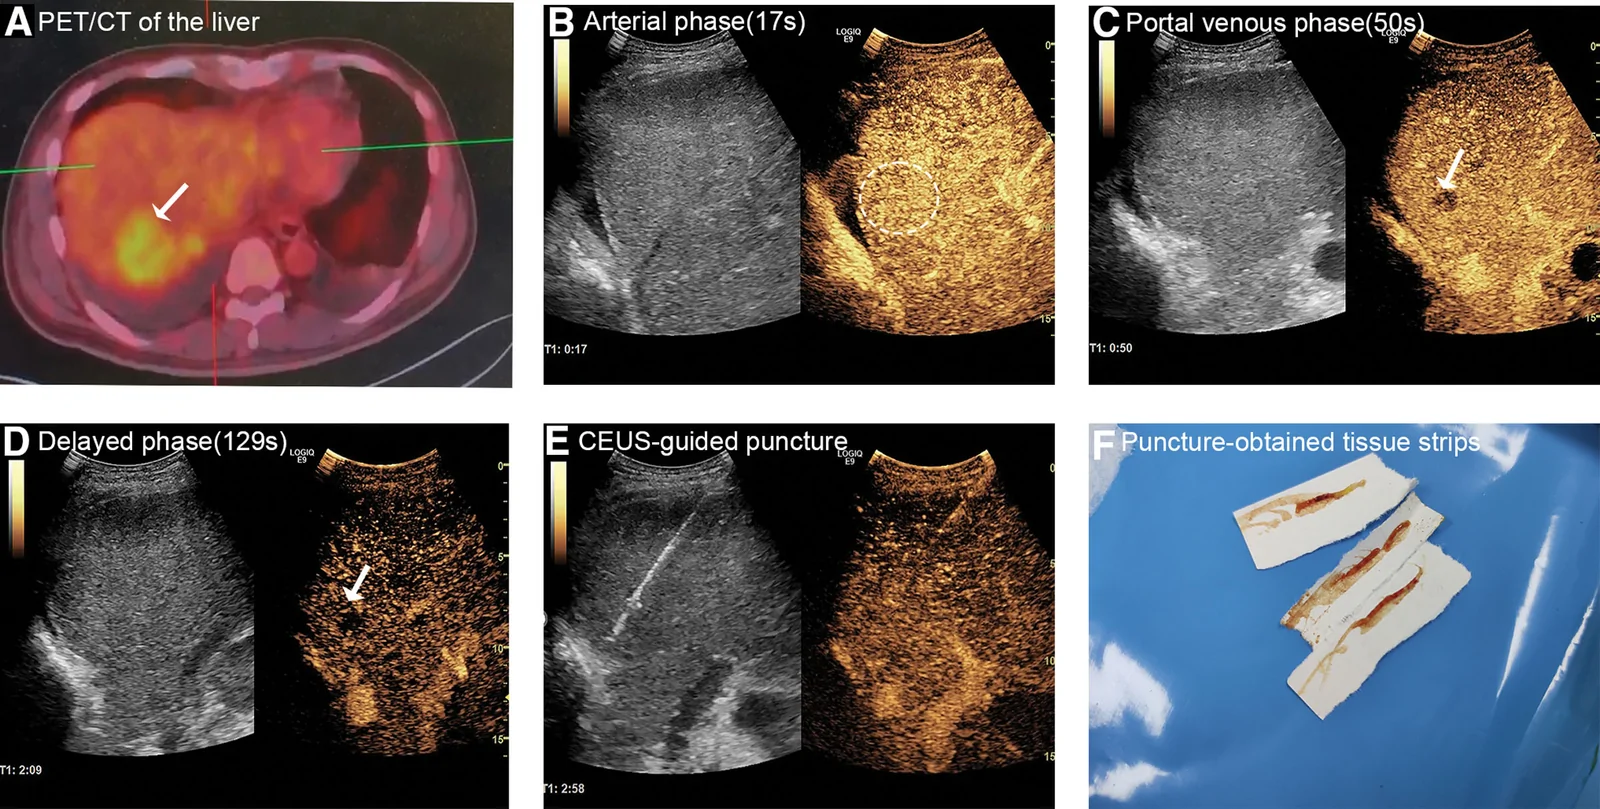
Liver lesion imaging and biopsy in a 41-year-old man with recurrent fever and melena. (A) PET-CT images showed liver uptake of FDG (arrow), suggesting multiple hepatic lesions suspicious for malignancy. (B–D) CEUS was performed with injection of 2.4 mL sulfur hexafluoride microbubbles (SonoVue; Bracco) at a low mechanical index (<0.12): (b) Arterial phase (17 seconds): the lesion showed iso-enhancement (circle); (C) Portal venous phase (50 seconds): the lesion showed hypo-enhancement (arrow); (D) Delayed phase (129 seconds): the lesion showed hypo-enhancement (arrow); (E) CEUS-GB was performed with an 18G automatic cutting biopsy needle after clear visualization of the lesion. (F) Three tissue strips were obtained and submitted for pathological and flow cytometry analysis. The patient was diagnosed with NK/T-cell lymphoma and hemophagocytic syndrome. CEUS = contrast-enhanced ultrasound; CEUS-GB = contrast-enhanced ultrasound-guided biopsy, FDG = 18F-fluorodeoxyglucose, PET-CT = positron emission tomography.
